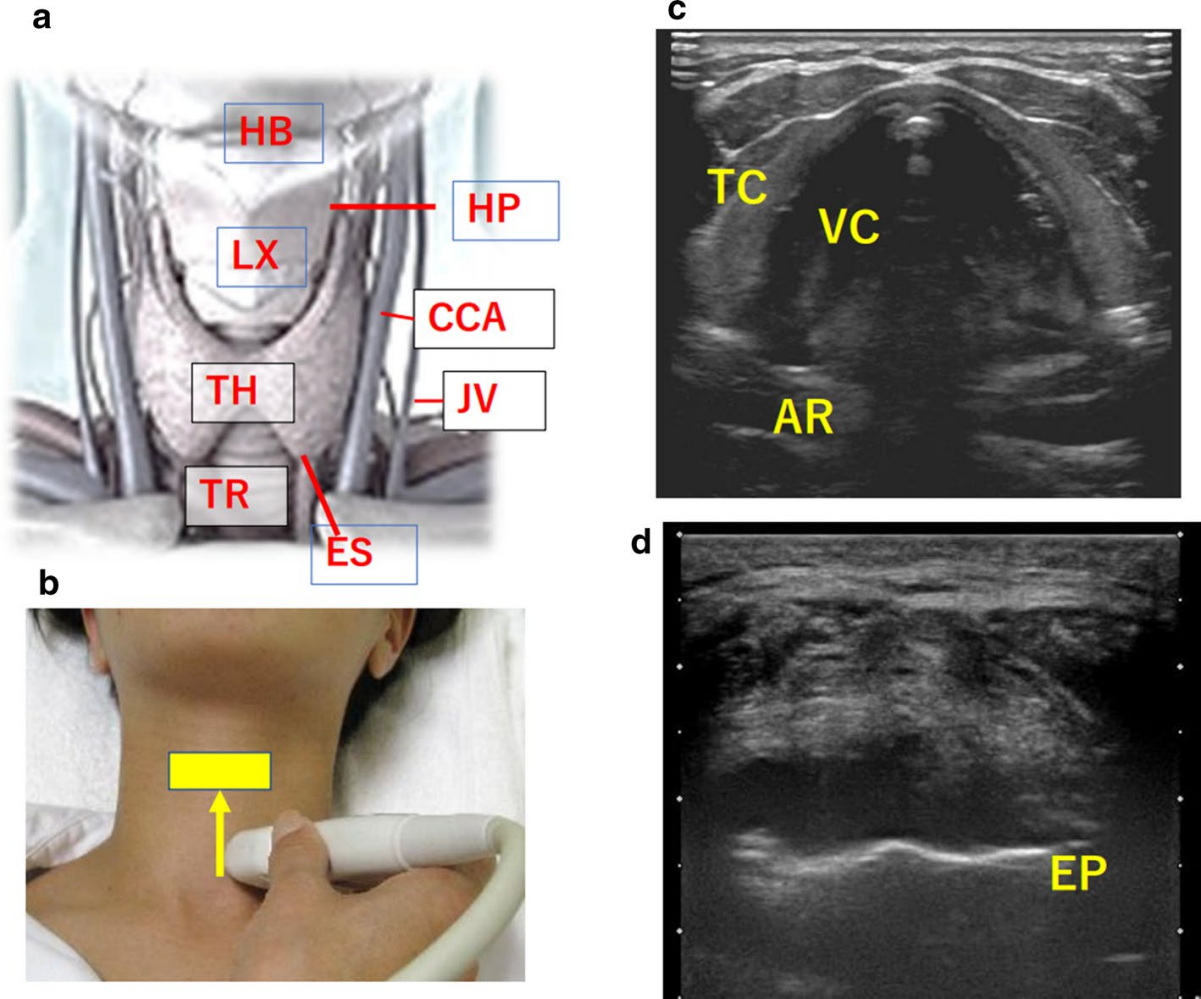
POCUS of the anterior neck *HB* hyoid bone, *LX* larynx, *TH* thyroid gland, *TR* trachea, *HP* hypopharynx, *ES* esophagus, *CCA* common carotid artery, *JV* internal jugular vein, *VC* vocal cord, *TC* thyroid cartilage, *AR* arytenoid cartilage, *EP* epiglottis cartilage. a Anterior neck anatomy. b Anterior neck probe operation. c Transverse view of the anterior neck: Ultrasound image of the larynx (vocal cord level). d Transverse view of the anterior neck: Ultrasound image of the larynx (supraglottic level)
